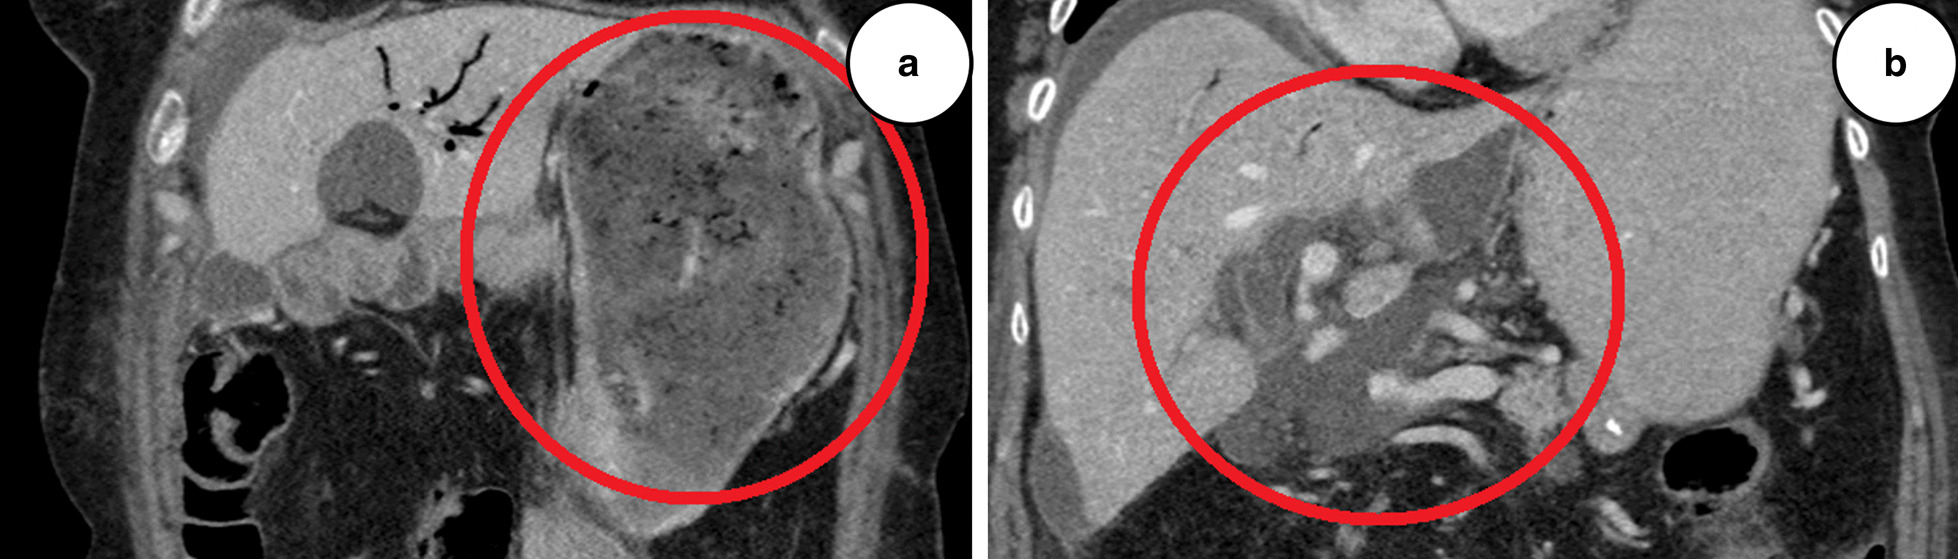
a Computed tomography, coronal section of the upper abdomen, postoperative day 10, showing gastric retention. b Computed tomography, coronal section of the upper abdomen, postoperative day 10, showing free low-density fluid around the liver and in the vicinity of hepaticojejunostomy, consistent with insufficient hepaticojejunostomy
